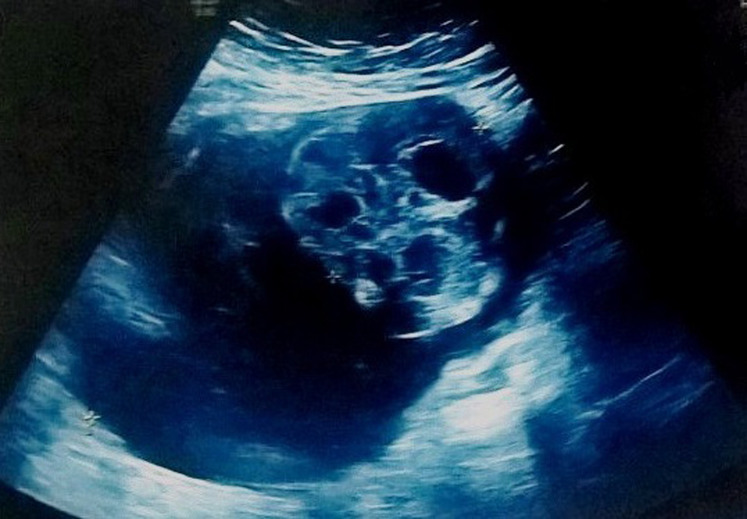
ultrasonography showing a right adnexal mass measuring 126 mm with a double tissue and cystic components
